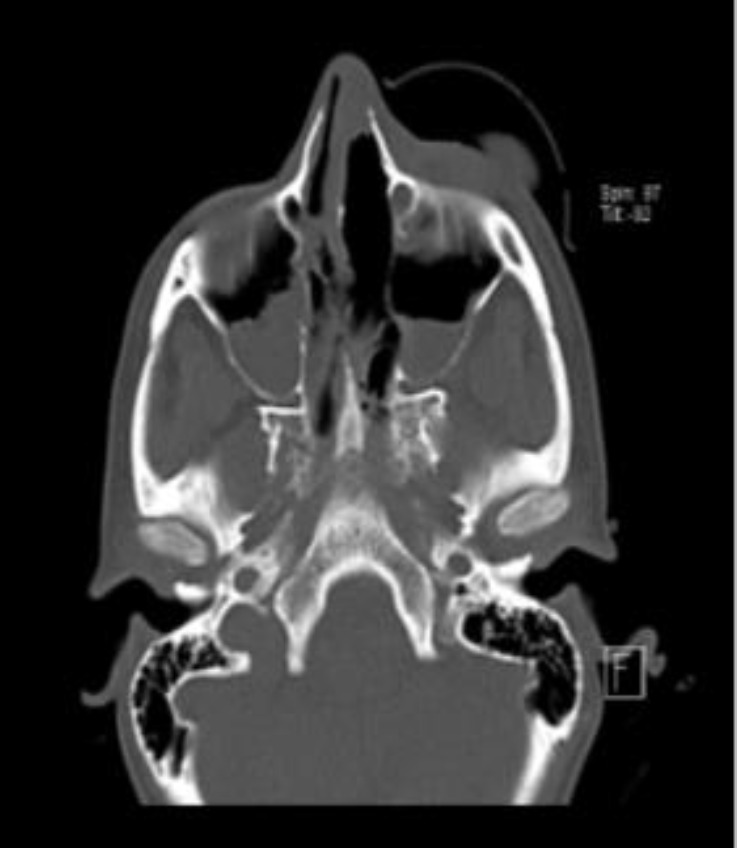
The PNS CT scan (axial view-without contrast) shows a mucosal thickening in both maxillary sinuses
